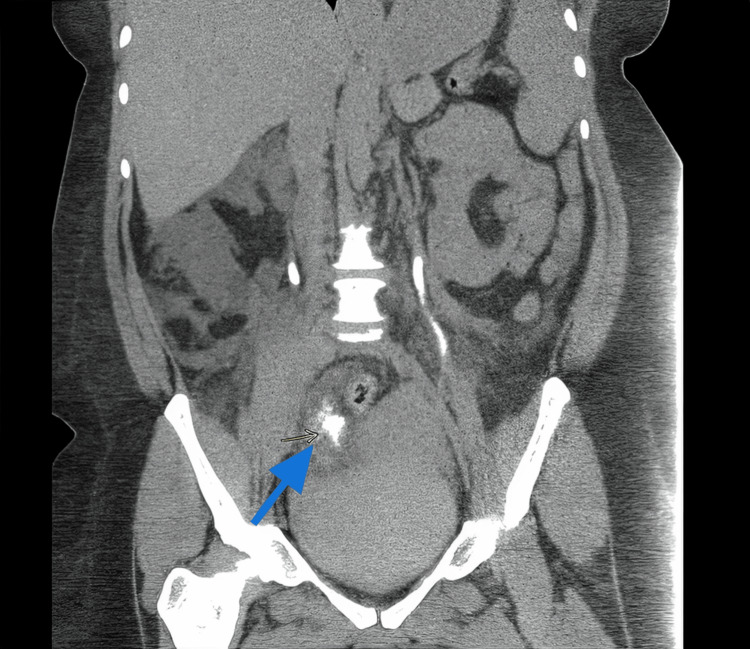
CT post-contrast delayed phase coronal image: showing extravasation of contrast near the distal right ureteric course (arrow). The left ureter appears normal in course and caliber.
